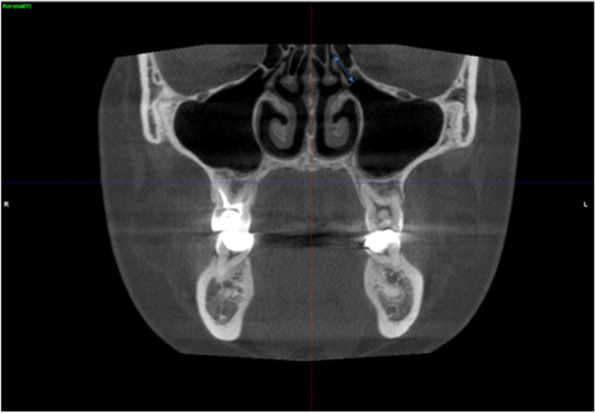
Coronal CBCT images showing the infundibulum length
